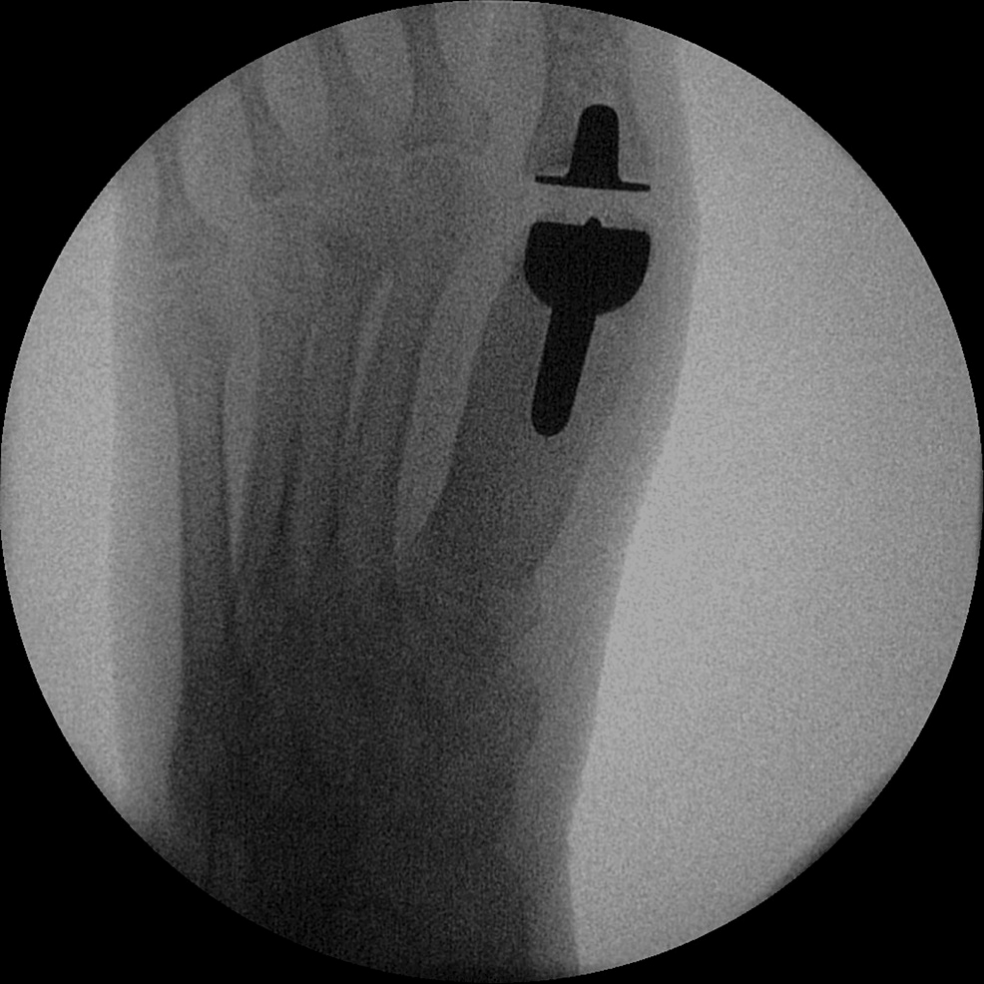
Intra-operative X-rays during first metatarsophalangeal joint replacement.
